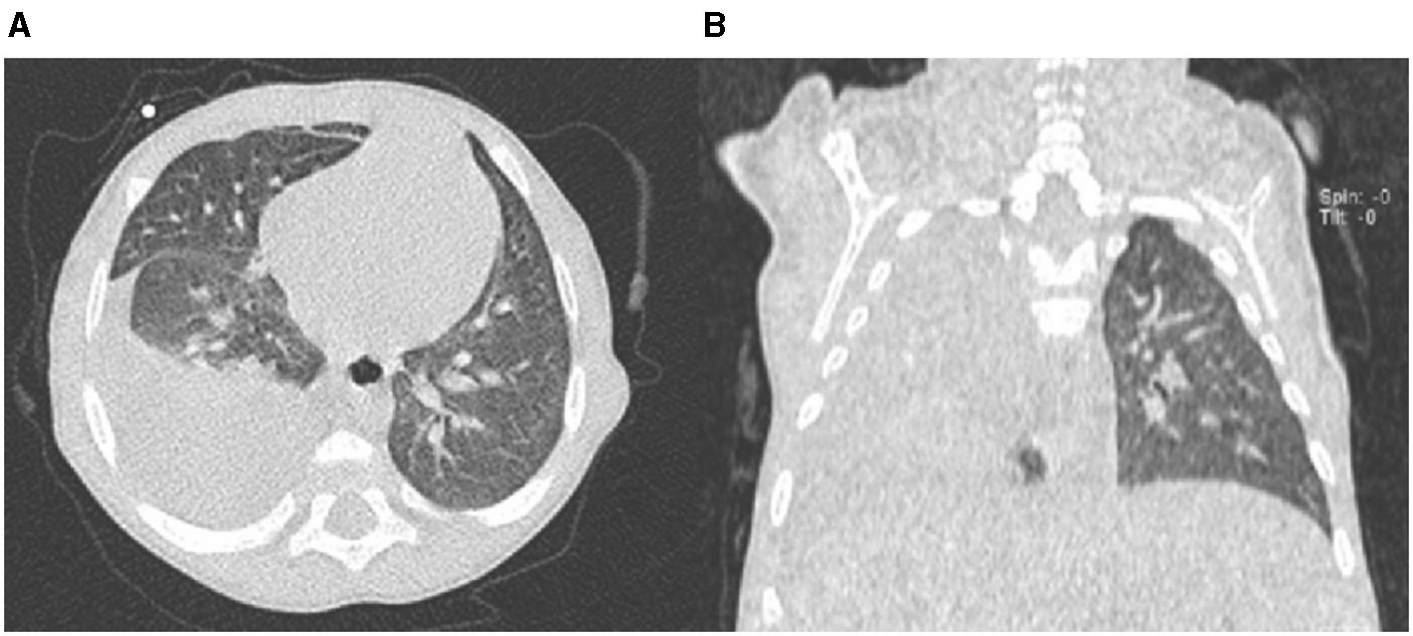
Chest computed tomography (CT) scan axial view (A) and coronal view (B) showing right-sided pleural effusion.
